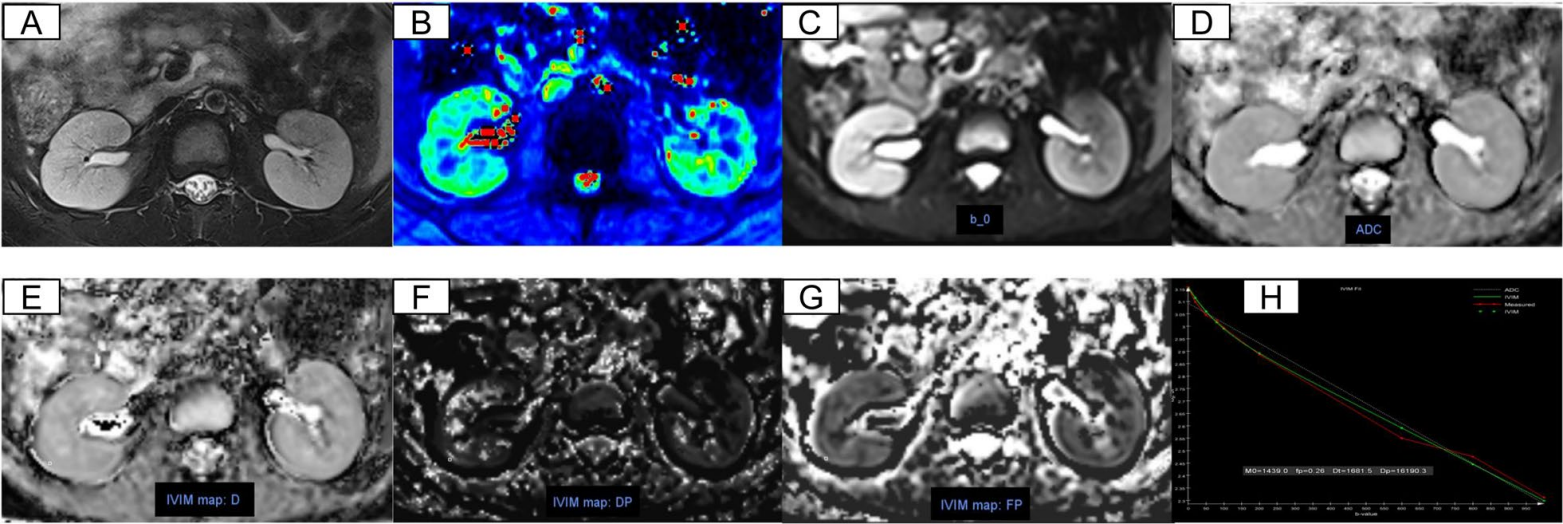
Respective MRI images of CKD stage 1–3 (7 years old, male). A–H Axial T2-weighted images, T2* mapping, b0 map, ADC, *D*_*t*_, *D*_*p*_, *f*_*p*_, IVIM fit, respectively
